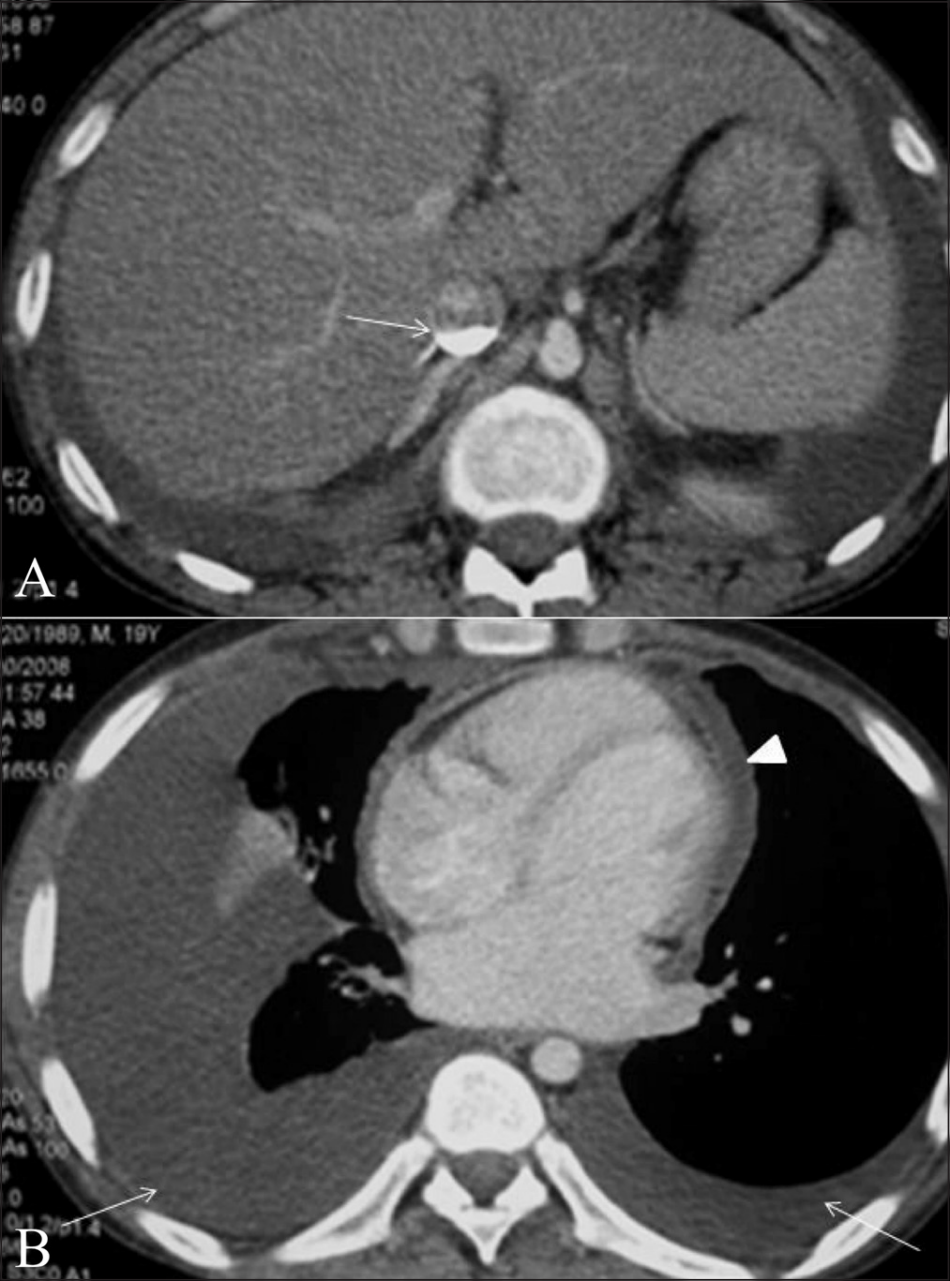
Contrast layering in the IVC (arrow in A) is seen on a contrast-enhanced, axial CT scan of a patient who died of cardiogenic shock within 24 h. He had bilateral pleural (arrows) as well as pericardial (arrowhead) effusions (B). No signs of cardiac tamponade are seen in the scan
